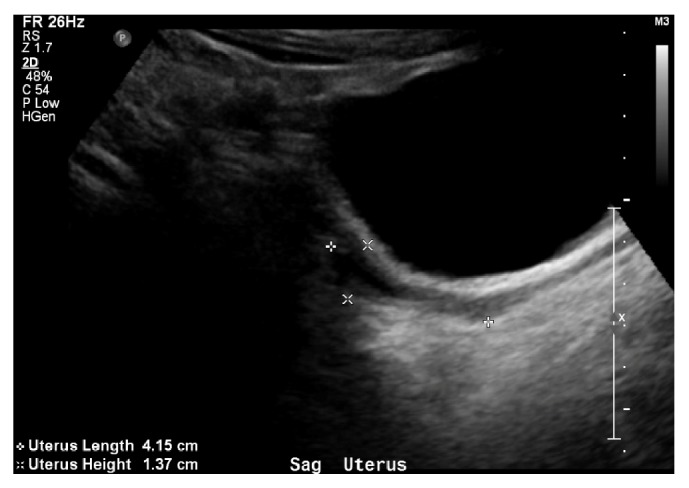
Transabdominal ultrasound of uterus.
